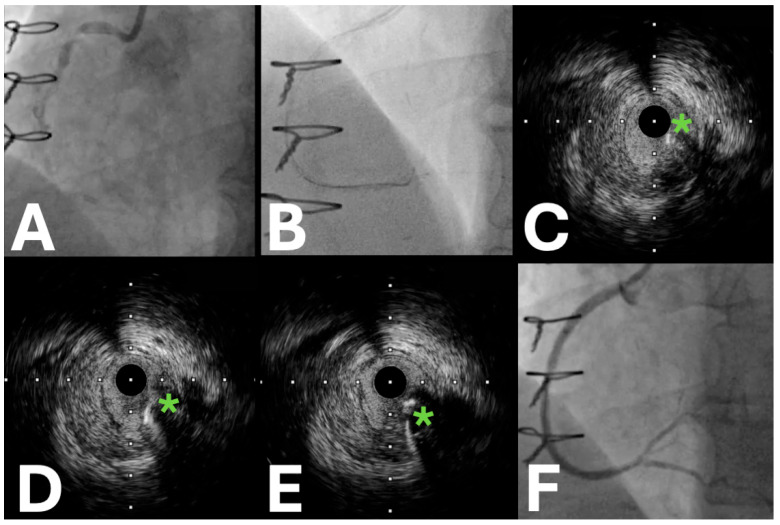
Intravascular ultrasound is used for the tip detection, antegrade dissection, and re-entry. (A) The baseline coronary angiography of a chronic total occlusion of the proximal right coronary artery. (B) The fluoroscopic image of the tip detection–antegrade dissection and re-entry under intravascular ultrasound guidance. (C) The shaft of the stiff guidewire on the intravascular ultrasound. (* indicates the guidewire position) (D,E) The tip of the stiff guidewire entering the true lumen on the intravascular ultrasound. (* indicates the guidewire tip position). (F) The final angiographic result after the stent implantation.
